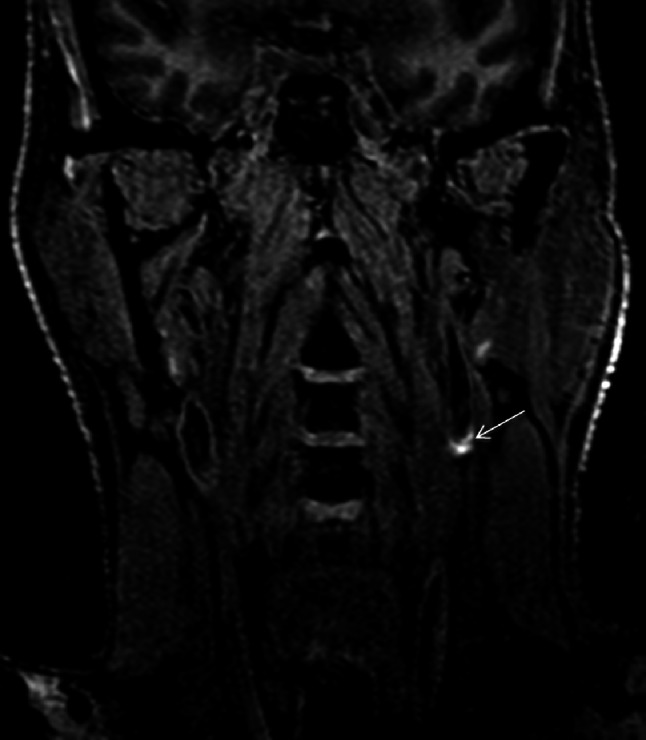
Coronal T1-weighted IR 3D FFE image depicting a hyperintensity in the left carotid artery indicating intraplaque hemorrhage. Hyperintense signal in the carotid wall >150 % of the adjacent sternocleidomastoid muscle on this sequence accurately and reliably depicts intraplaque hemorrhage
